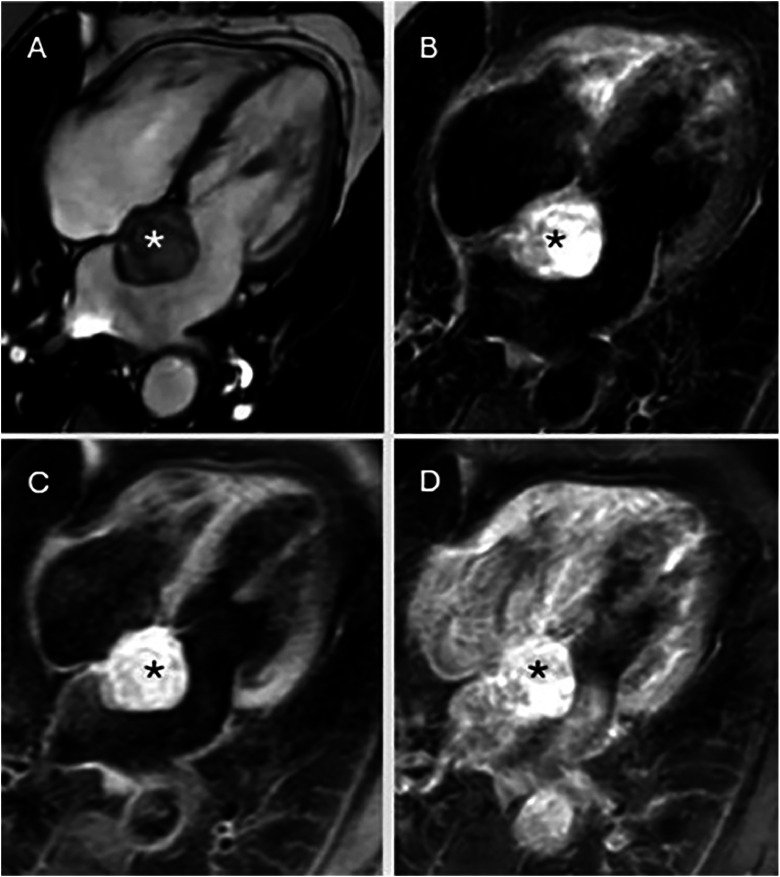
Cardiac magnetic resonance (CMR) images: (A) SSFP 4-chamber view showing the mass within the left atrium (*). (B) T2 STIR, 4-chamber view demonstrating high T2 signal within the tumor, suggesting edema or increased water content. (C) T1-weighted sequence without contrast showing high heterogeneous signal intensity within the tumor. (D) T1-weighted sequence with contrast showing slightly diffuse inhomogeneous gadolinium enhancement.
